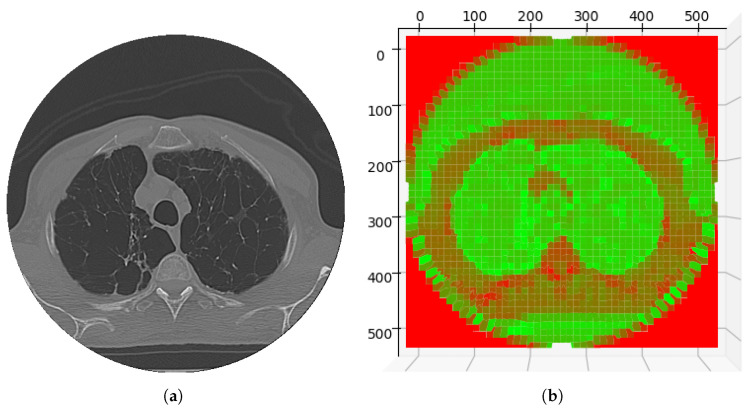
CAF energy map for sample lung CT image. (a) Input image. (b) External energy CAF.
